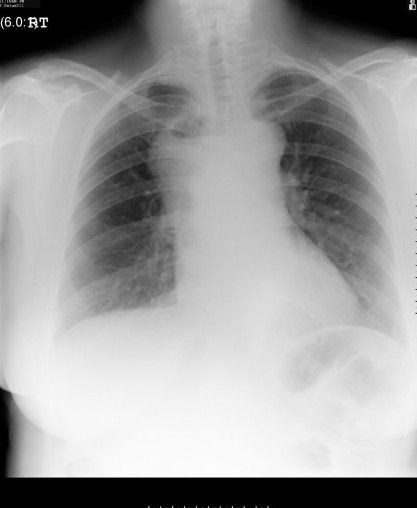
Chest radiograph showing the cystic mass, now with an air-fluid level (compare Fig 1).
